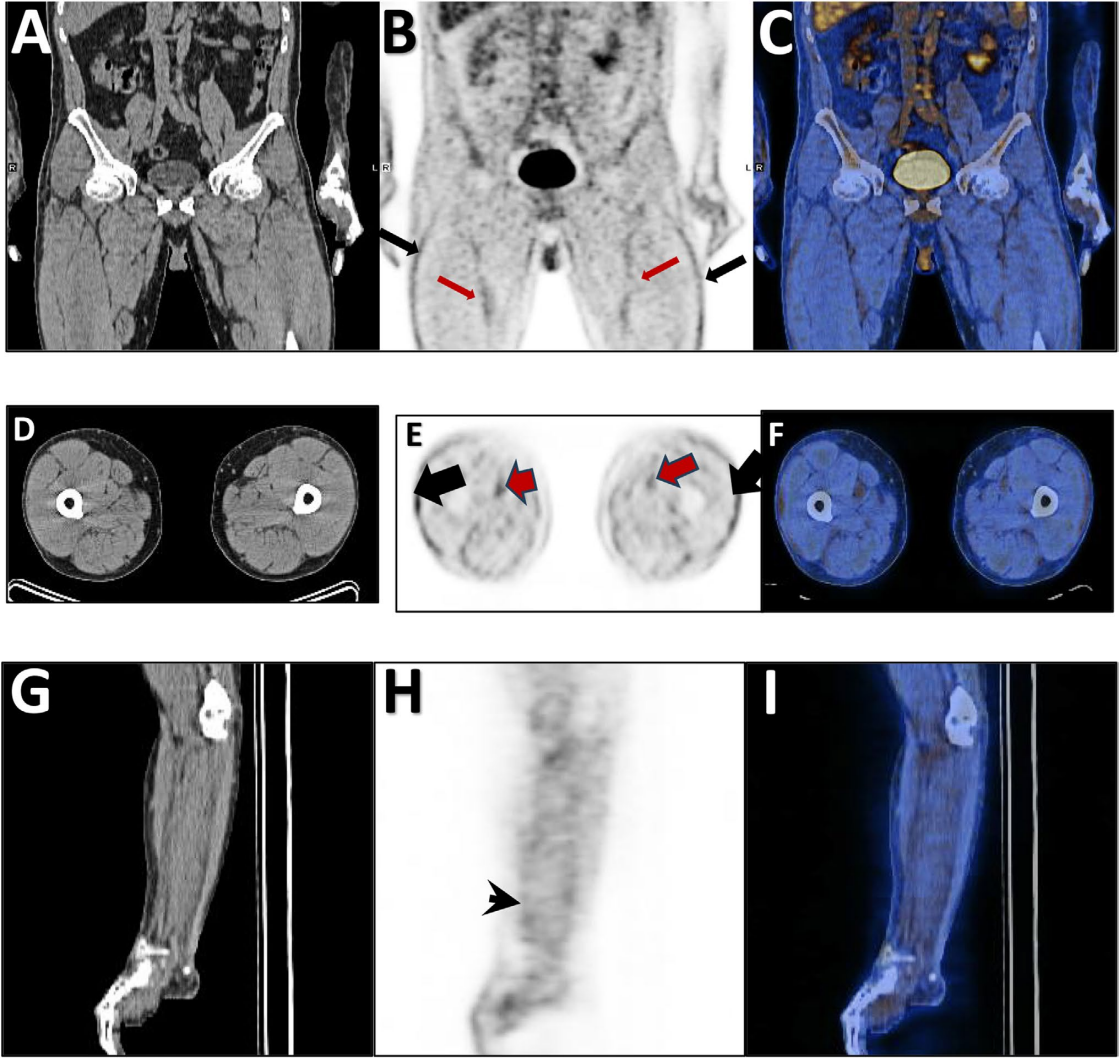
The 18F‐FDG PET/CT scan in coronal and axial views showed bilateral fascia‐lata fasciitis (black arrow in B, E); as well as intermuscular fasciitis (red arrow in B, E) (A‐F). Also, the PET/CT scan revealed medial and lateral intermuscular fasciitis (black arrow‐head in H, G‐I).
